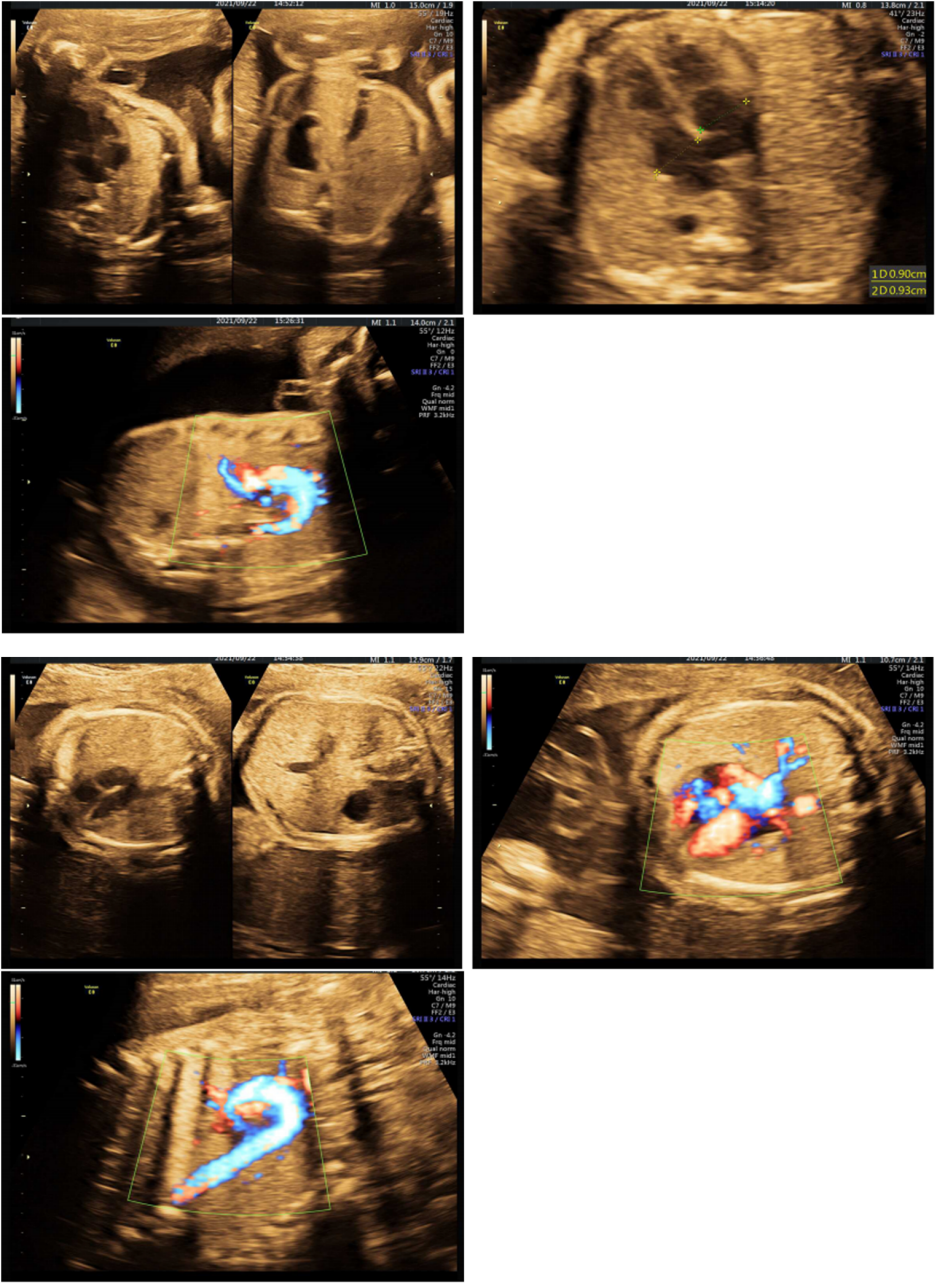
Doppler echocardiography of two fetuses.
